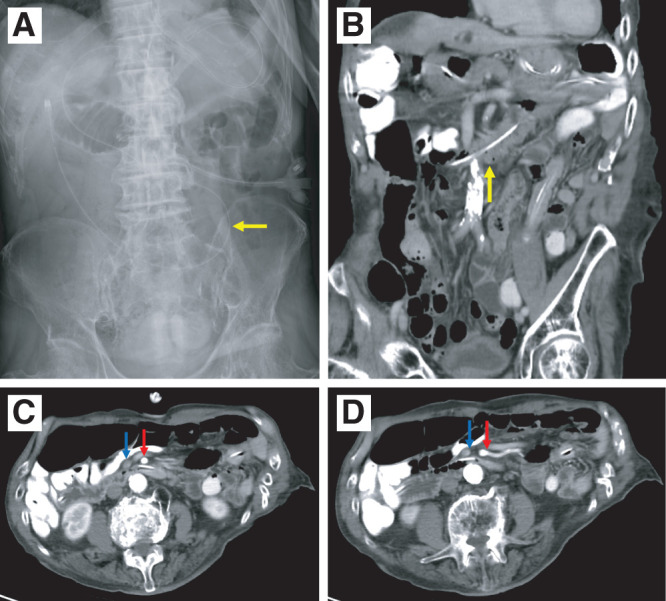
Imaging findings after spontaneous resolution of small bowel volvulus. Anteroposterior abdominal X-ray (A) and abdominal multidetector CT images—coronal section (B), and axial sections at the L2 vertebral level (C) and L4 vertebral level (D). A percutaneous endoscopic gastrojejunostomy tube is in place. The third portion of the duodenum (yellow arrow) traversed between the AA and the SMA from right to left, and the duodenojejunal junction had naturally relocated to the left abdomen. At both the L2 vertebral level and the L4 vertebral level, the SMV (blue arrow) was located to the right of the SMA (red arrow). AA, abdominal aorta; SMA, superior mesenteric artery; SMV, superior mesenteric vein
